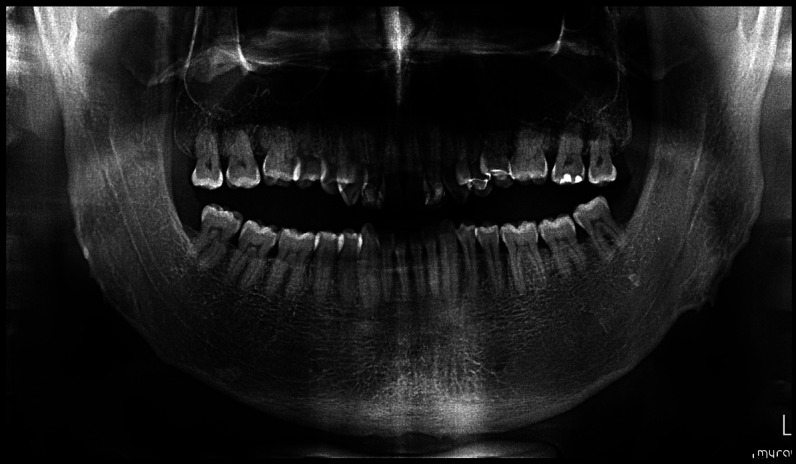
Panoramic radiographic view of the patient in June 2024.
